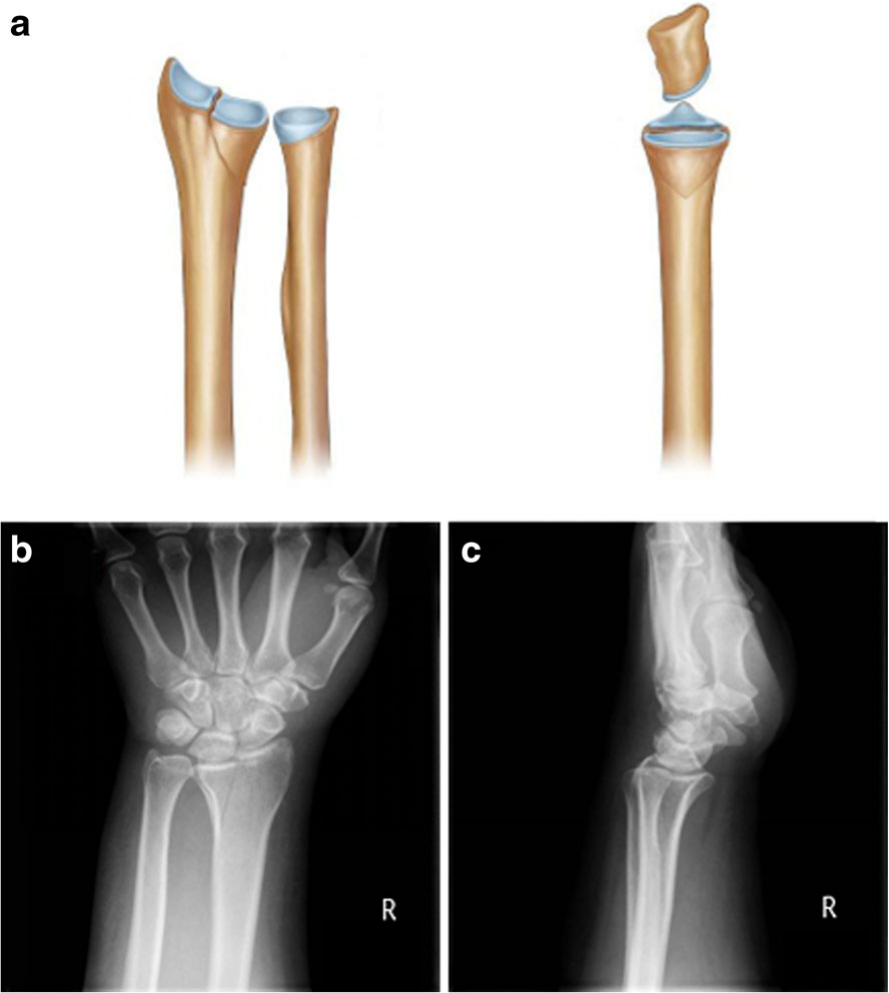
Vertical depression fracture. a The entire lunate fossa is vertically depressed (*left*, anterior view; *right*, lateral view). b, c Anteroposterior and lateral radiographs (right)
